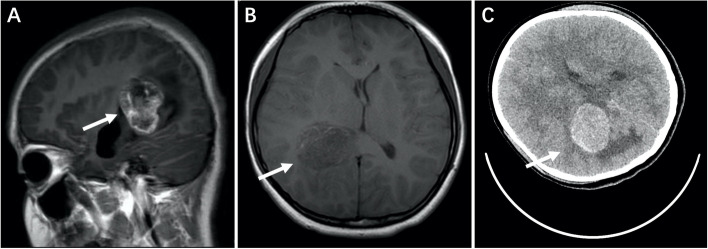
Brain MRI (A, B) and CT (C) in the Emergency Department before the operation. High-density space-occupying lesions were identified in the right temporal parietal lobe and the size was approximately 4cm*5cm*3cm
